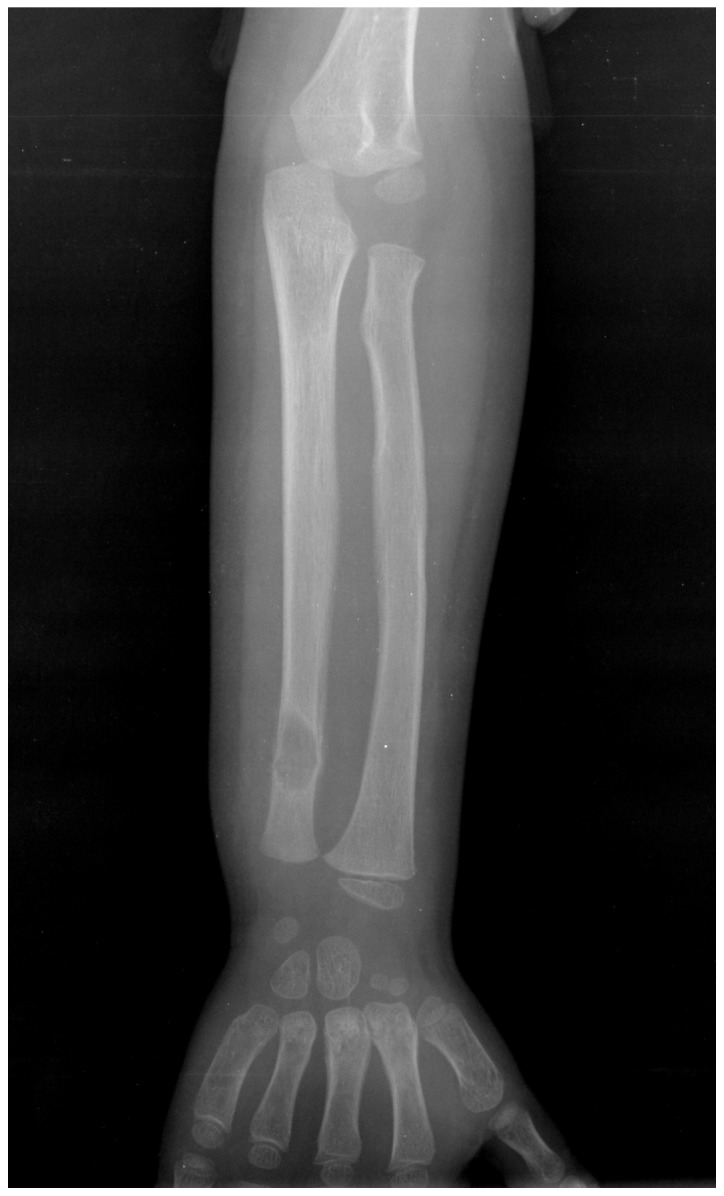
Case 1. X-ray demonstrating a well-circumscribed osteolytic lesion with slight marginal sclerosis involving the diaphysis of the distal left ulna.
